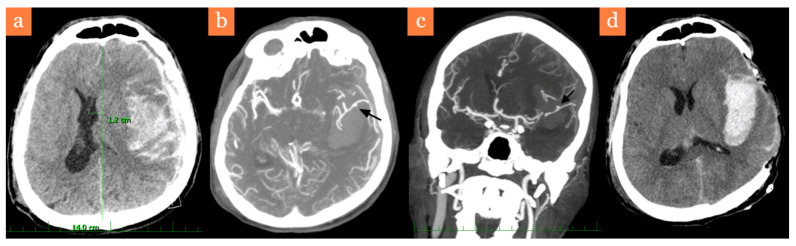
Graphic depiction of the case before the second surgery. (a) CT performed immediately after admission, showing a severe midline shift of 12 mm, (b,c) axial and coronal CT angiography (CTA) views depicting a large intracerebral hematoma in the sylvian fissure and a broad base M3 aneurysm, (d) postoperative CT after decompressive craniectomy (DC), without complications with midline shift regression and the intact intracerebral hematoma.
